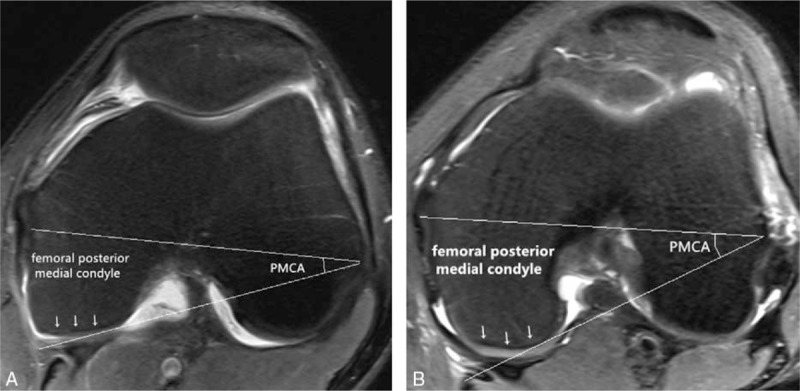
MRI images of the patient (A) and a normal knee (B) in the axial plane. The posterior medial condyle angle (PMCA) of the current patient was smaller than normal. The articular surface of the posterior medial condyle is obviously flatter than in the normal knee. These findings suggest hypoplasia of the femoral posterior medial condyle. MRI = magnetic resonance imaging.
